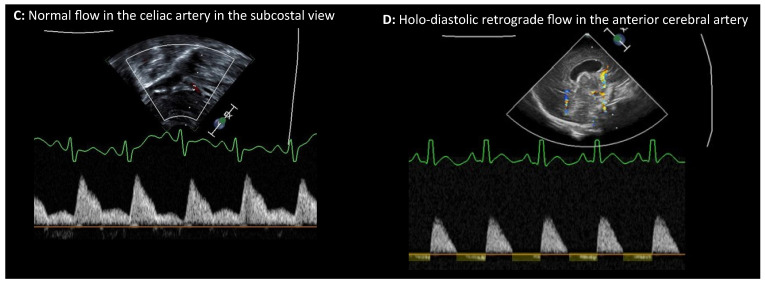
Doppler Flow Patterns in the Celiac and Cerebral Arteries (End-Organs) in the context of a “Diastolic Steal Effect” by the Left-to-Right PDA Shunt. (A) Holo-diastolic retrograde flow in the celiac artery obtained by PW Doppler in the subcostal view. (B) Absent end-diastolic flow in the celiac artery obtained by Doppler in the subcostal view. (C) Holo-diastolic retrograde flow in the anterior cerebral artery in the sagittal transfontanel views. (D) Normal antegrade diastolic flow in the celiac artery in the subcostal view serving as a reference pattern in a hemodynamically stable neonate.
